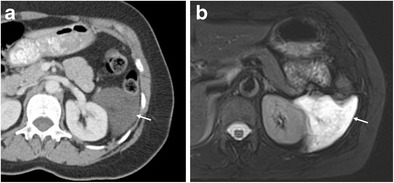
a A well-defined and fluid attenuation homogeneous cystic lymphangioma in the left perirenal space (*arrow*), with no capsule and no evidence of mass effect on the adjacent kidney. b A T2-weighted MRI shows the fluid signal of the lesion (*arrow*)
